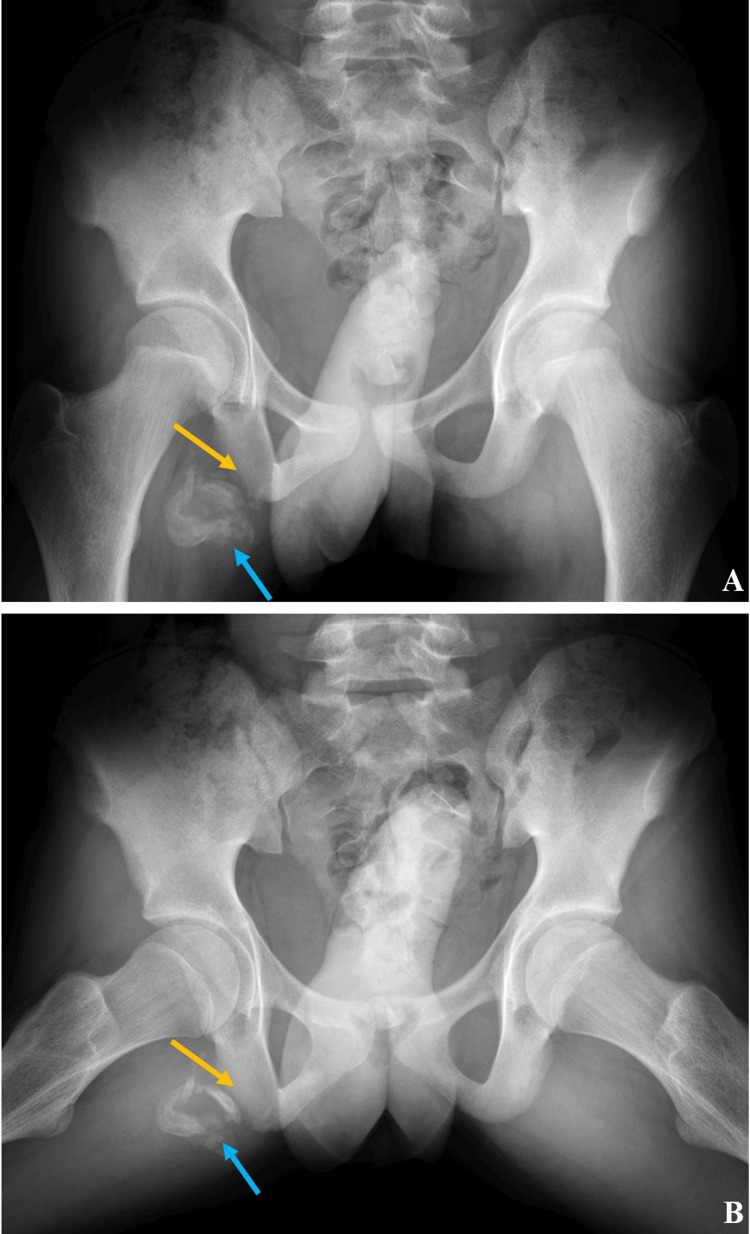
Radiographs of bilateral hips AP (Figure 1A) and frog leg (Figure 1B) radiographs demonstrate lucency and irregularity of right ischial tuberosity suggesting avulsion fracture (yellow arrows). There is a cluster of well-corticated, ovoid, and crescent-shaped calcifications in the soft tissues near the dominant fracture fragment (blue arrows).
